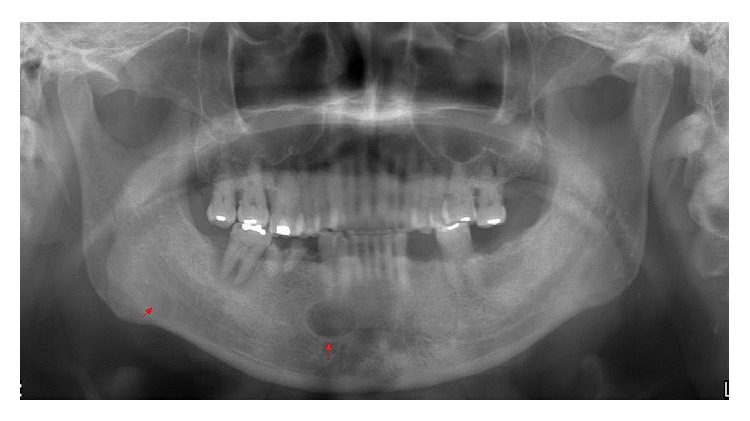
Preoperative panoramic radiography shows two regions of radiolucency in the right mandible, as a well-defined, oval radiolucency just inferior to the premolar root apex, and a slight oval radiolucency at the angle of the mandible below the inferior alveolar canal.
